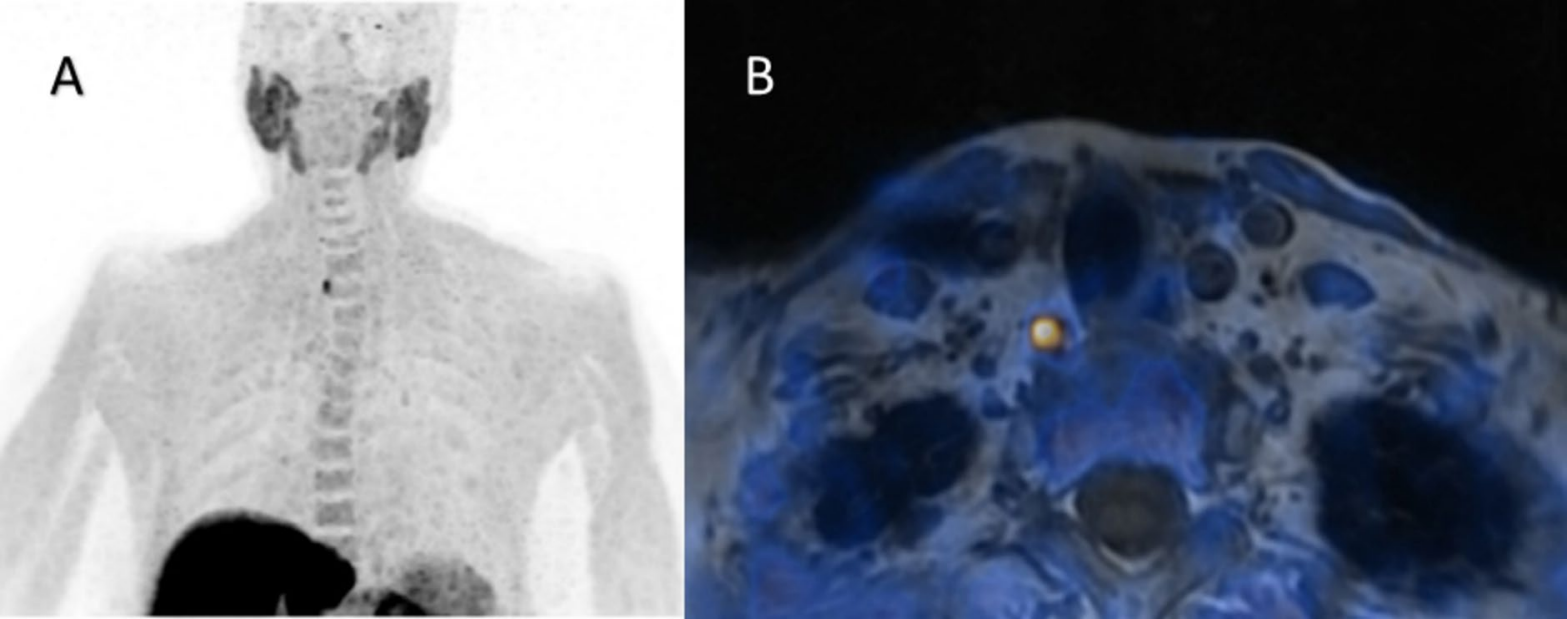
Overly descended right-sided parathyroid adenoma. Legend: 75-year-old woman with pHPT and a history of relapsing kidney stones as well as osteoporotic fractures, albumin-corrected serum calcium level 2.65 mmol/L (2.19 – 2.54 mmol/L), PTH 141.0 ng/L (15-65 ng/L). MIP (A) and T1w-weighted axial PET/MR (B) image show an overly descended right-sided upper pole parathyroid adenoma (SUVmax 10.8, 11x6x6 mm), confirmed by surgery
